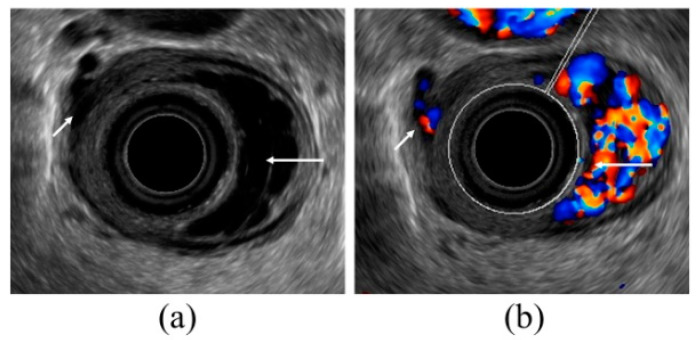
Observation of esophageal varices using a radial-arrayed echoendoscope (FUJIFILM, EG-580UR). (a) Observation in B-mode. Perforating veins (short arrow) and esophageal varix (long arrow) are observed. (b) Color Doppler observation. The esophageal varix (long arrow) is seen as a flow of mixed red and blue signals. Furthermore, the perforating vein (short arrow) is primarily presented in blue, and this site acts as the outflow passage from esophageal varices.
